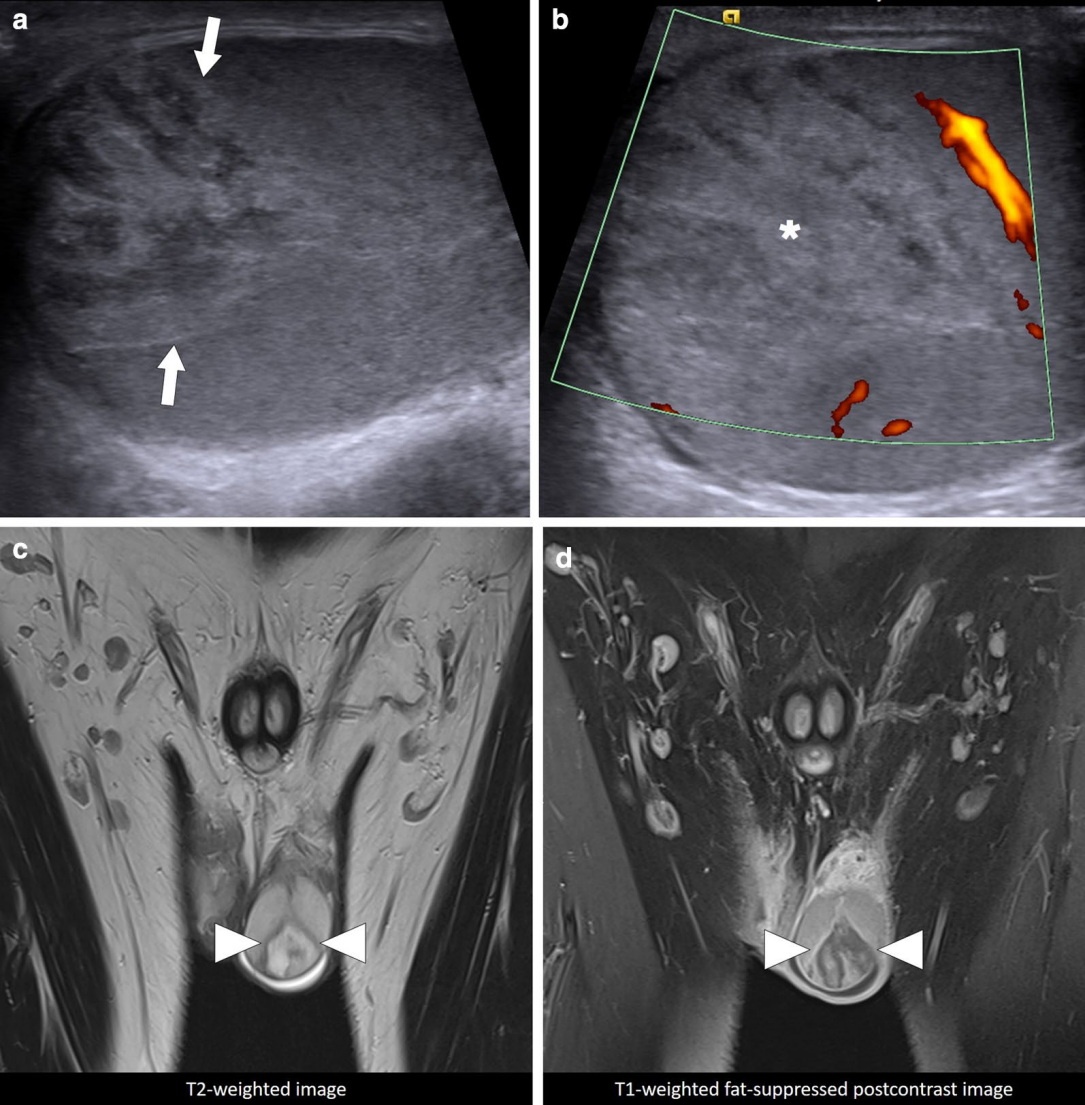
Segmental testicular infarction. A 32-year-old male with known Behcet’s disease presenting to the ER with left testicular pain. There was no history of trauma. a Gray scale US shows a triangular heterogeneous hyperechoic lesion (arrows) within the left testis. b On Power mode Doppler US, there was no vascularity within this focal area (asterisk). c Coronal plane T2W MR image shows triangular heterogeneous hyperintense lesion with a T2 hypointense rim. d Coronal plane postcontrast MR image demonstrates a hypoenhancing, wedge-shaped parenchymal area with sharp borders. Imaging findings were considered to be consistent with segmental testicular infarction, likely related to Behcet’s disease. Patient was placed on supportive treatment, to which he responded well. Serial follow-up US examinations revealed almost-complete resolution within 3 weeks after the initial event
